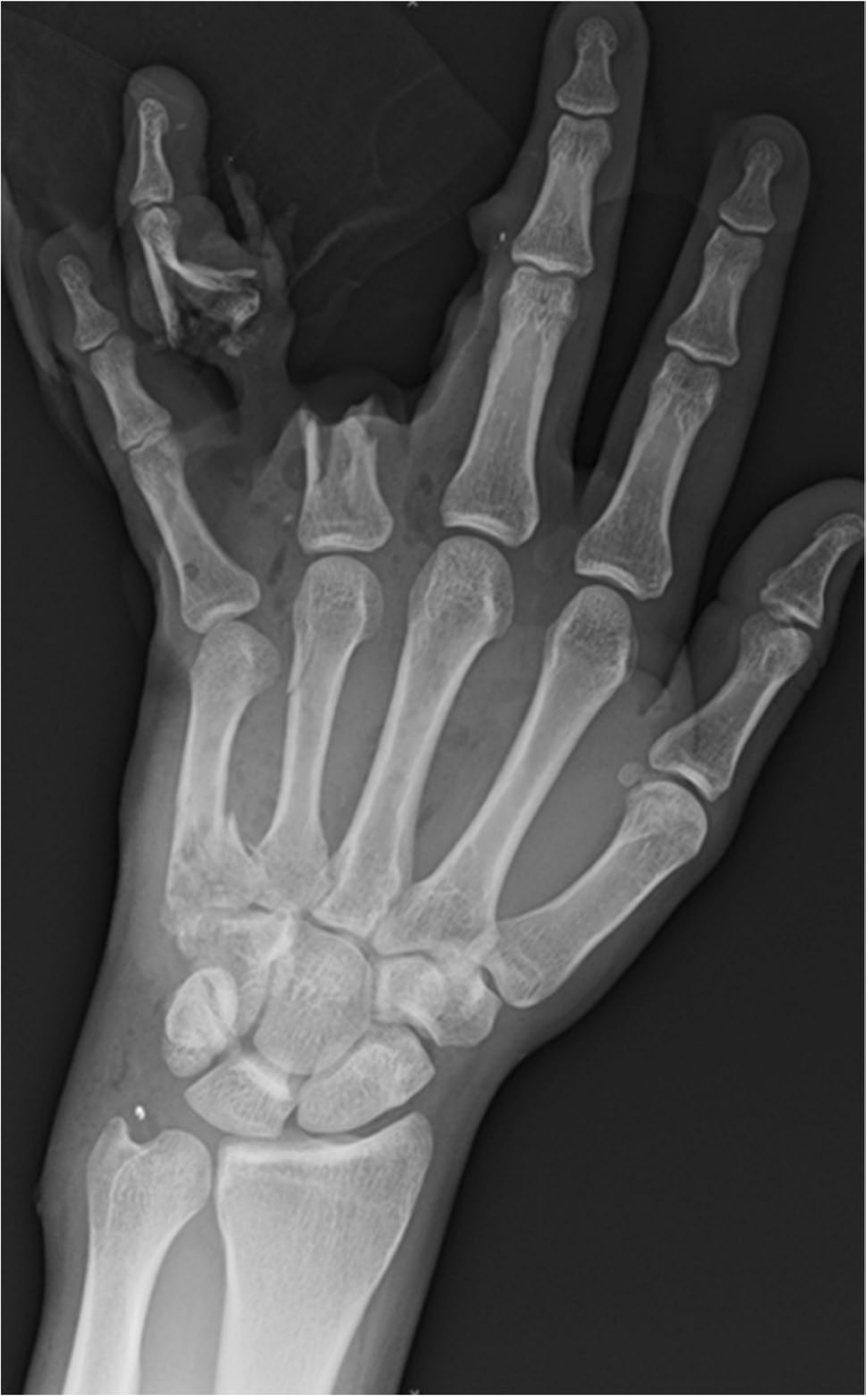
23-year-old male who presented with a gunshot wound to the left hand. Other than the amputation of the fourth finger and multiple fractures, the X-ray demonstrates small retained metallic bullet fragments about the 3rd proximal interphalangeal joint and ulnar styloid process
